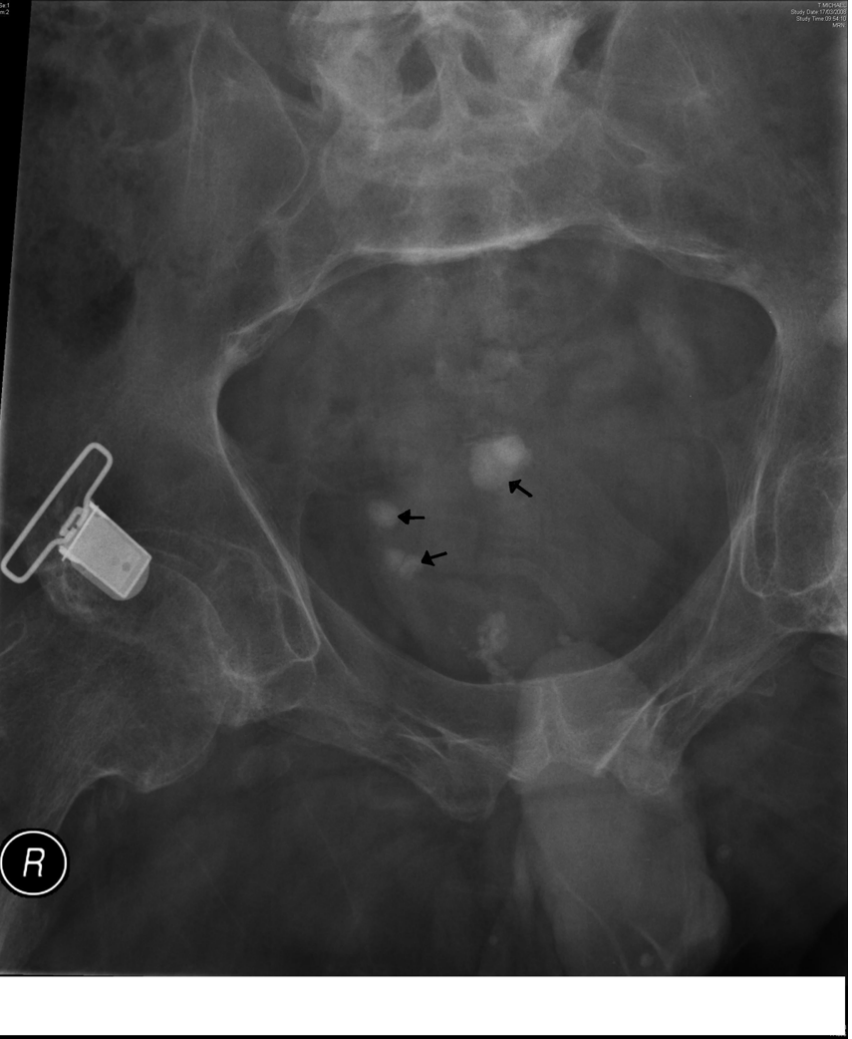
X-ray of urinary bladder (17 March 2008) shows four or five small stones (arrow).
